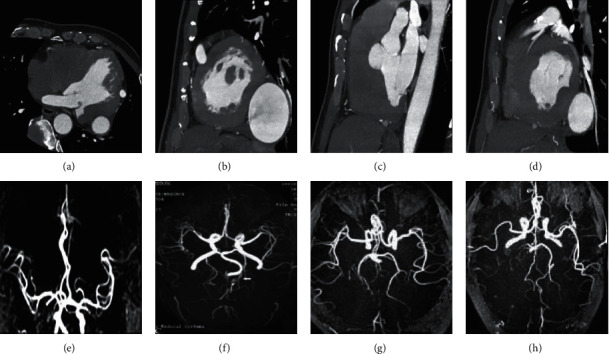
Coronary angiography images. (a–d) CTA images of four patients; (e–h)
